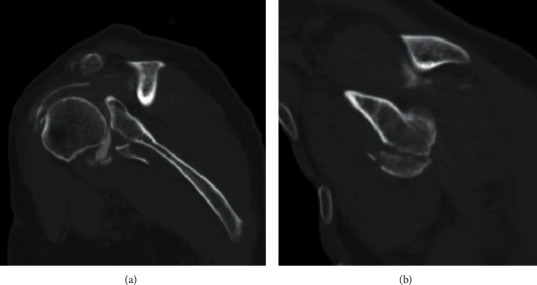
Coronal (a) and sagittal (b) CT images demonstrating comminuted bony Bankart fracture involving approximately 35% of the glenoid width.
